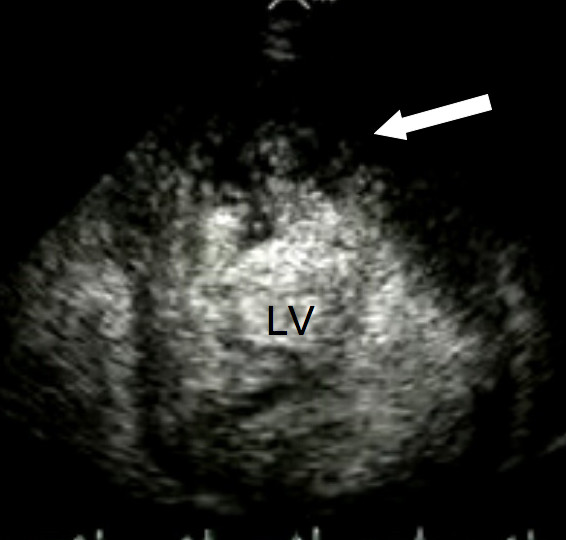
Transthoracic echocardiography. Following intravenous injection, contrast agent is visualised between the ventricular trabeculations (arrow). LV, left ventricle.
